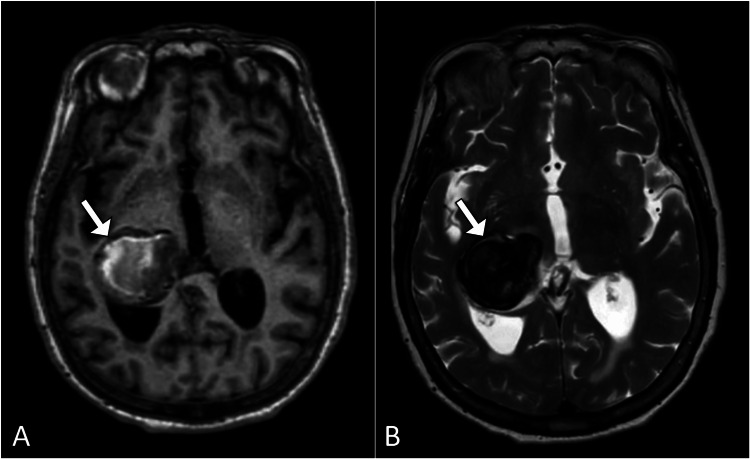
Axial brain magnetic resonance images (A) T1-weighted and (B) T2-weighted displaying an extra-axial hemorrhage (denoted by white arrows) located adjacent to the occipital horn of the right lateral ventricle.
